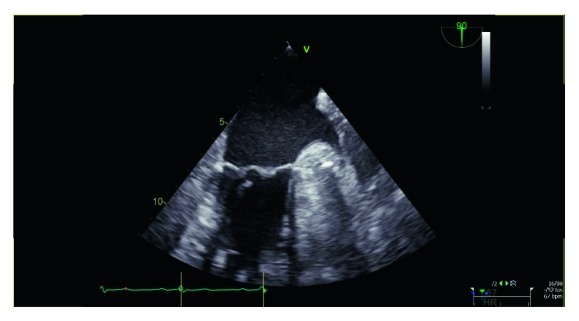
Transesophageal echocardiogram showing 0.9–1 centimeter mitral valvular vegetation.
